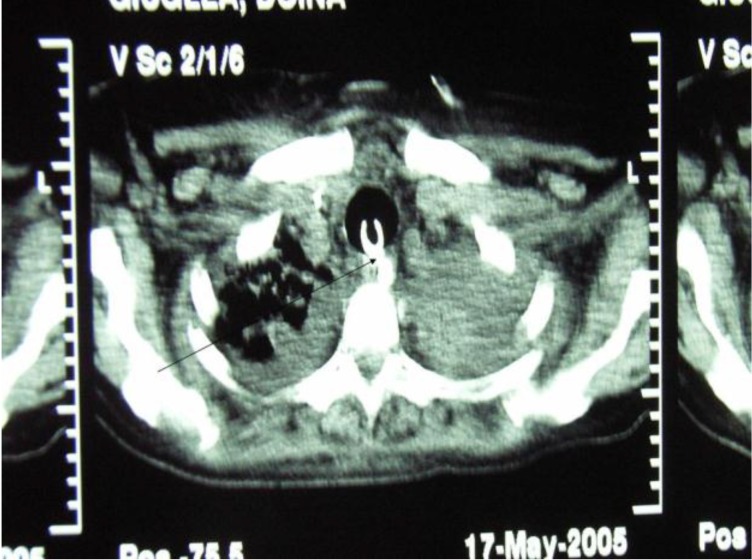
Preoperative CT scan – revealed the eso-tracheal fistula
